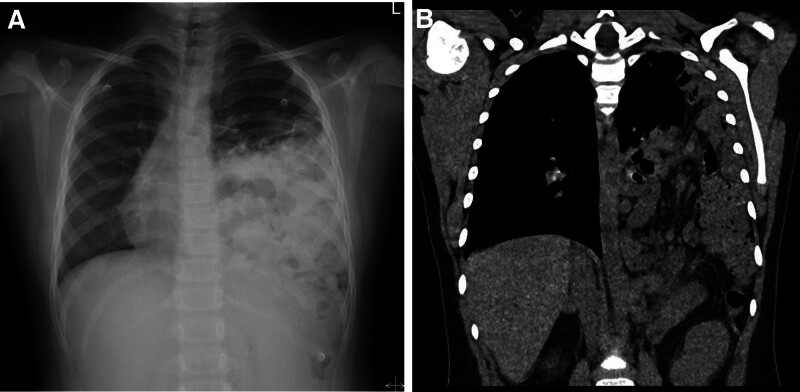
Preoperative imaging in case 2. (A) Chest x-ray shows a hazy shadow in the lower left chest cavity. (B) CT scan reveals a bowel loop herniated into the left thoracic cavity. CT = computed tomography.
